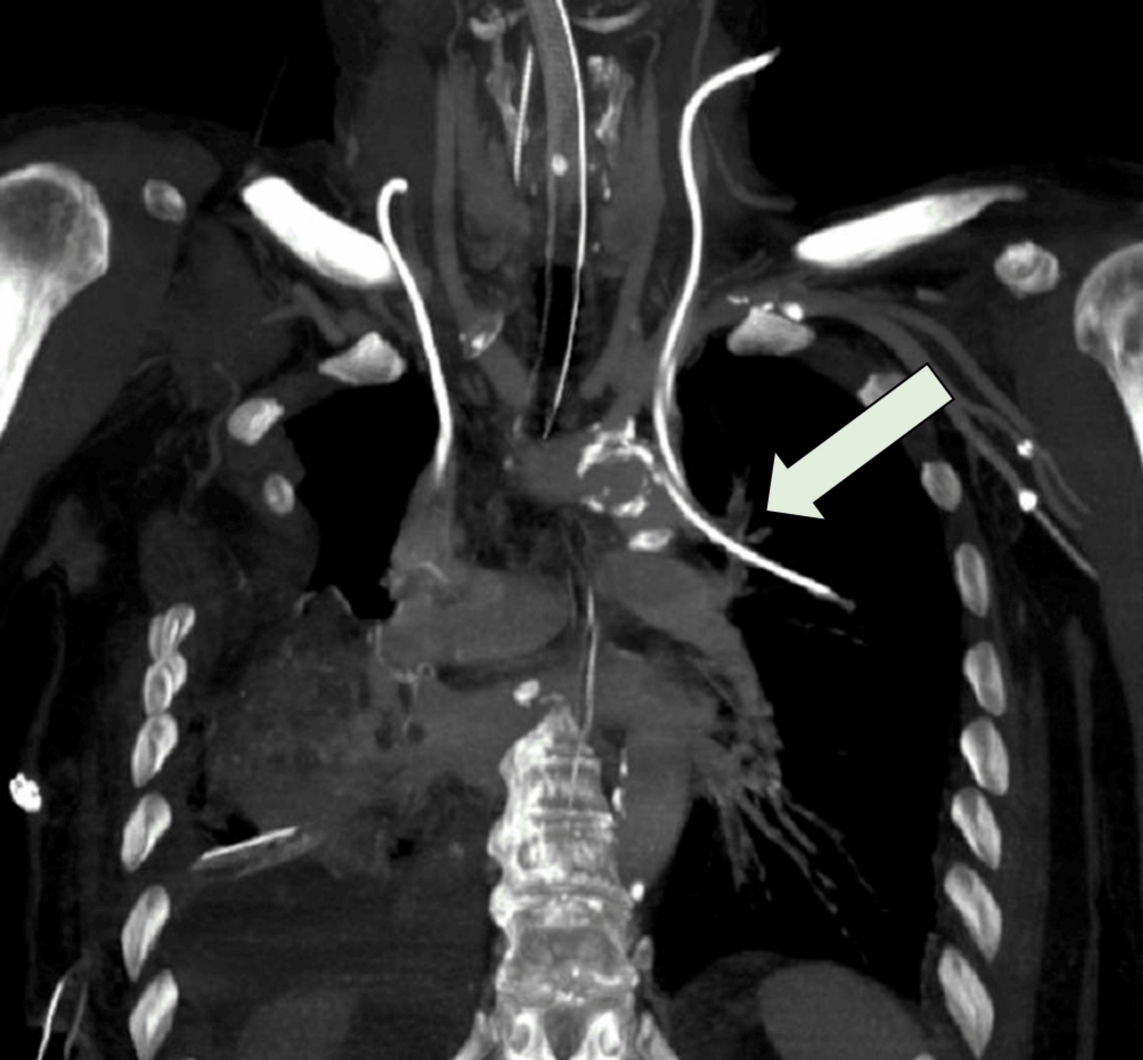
Coronal reconstruction from contrast-enhanced computed tomography angiography showing an anomalous vascular connection between the left upper pulmonary vein and the left internal jugular vein (arrow). The central venous catheter is visible within the anomalous vessel, highlighting the inadvertent placement in a pulmonary vein draining oxygenated blood into the systemic venous system.
